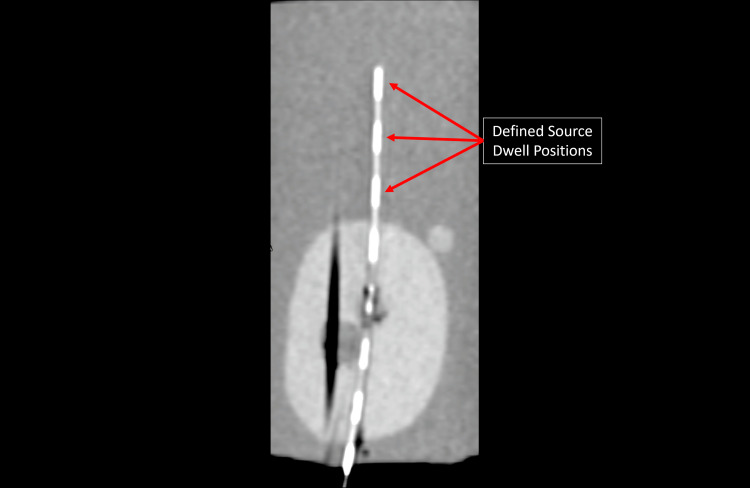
Sagittal plane of a CT image of a prototype marker designed to have discrete contrast regions. Each region is 1 cm long with a spacing of 1 cm between each region. The length of the contrast regions and the spacing between them is customizable and readily observable on CT images.
